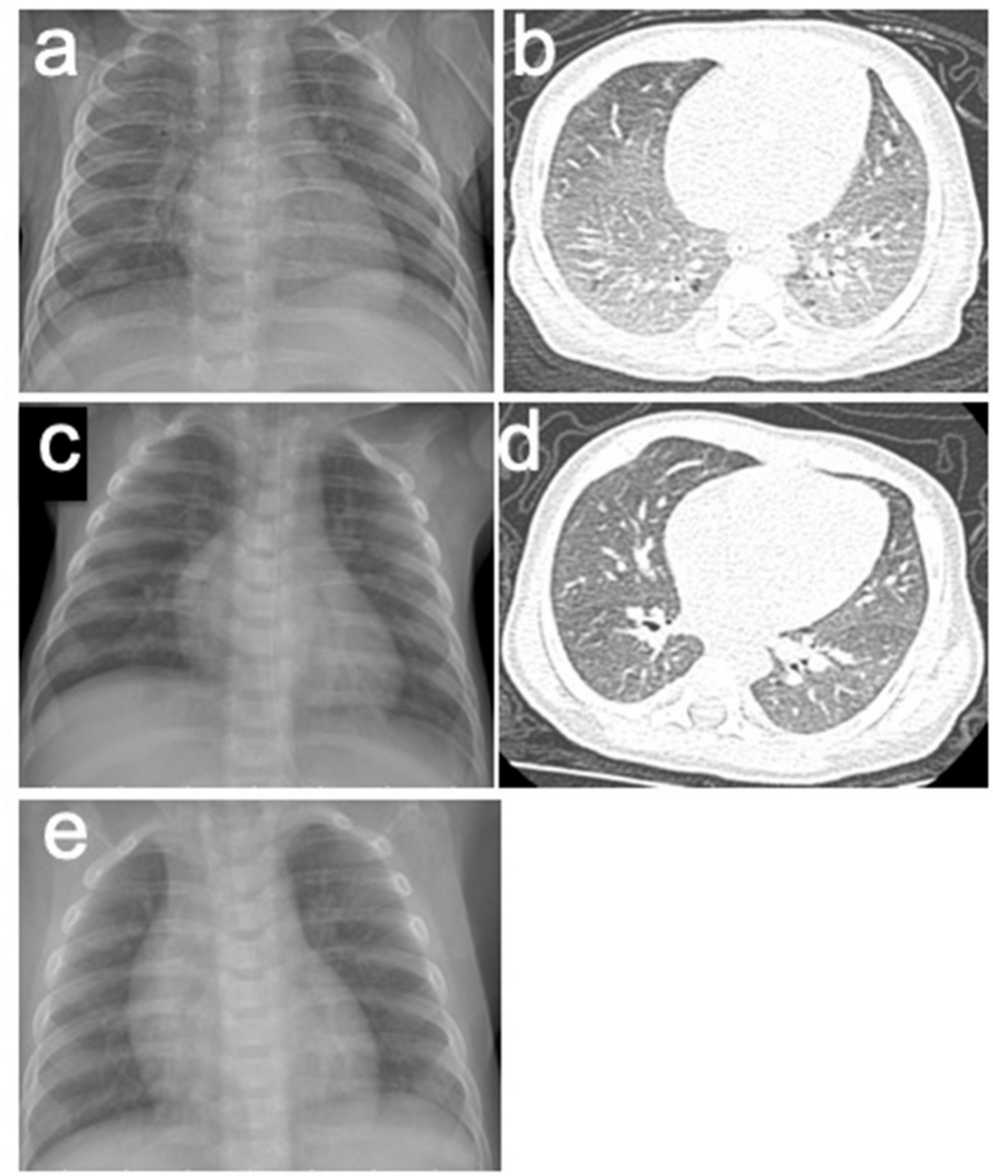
(a,b) X-rays and CT scans of the patient before the treatment of HS. (a) Chest roentgenogram showed bilateral infiltrates and opacities in different lung lobes. (b) Chest CT scan showed interstitial lung disease. (c,d) X-rays and CT scans of the patient after the treatment of HS. (c) Chest roentgenogram showed that pulmonary infiltrates cleared. (d) Chest CT scan was normal. (e) Chest roentgenograms in regular follow-up visit showed no infiltrates and opacities.
